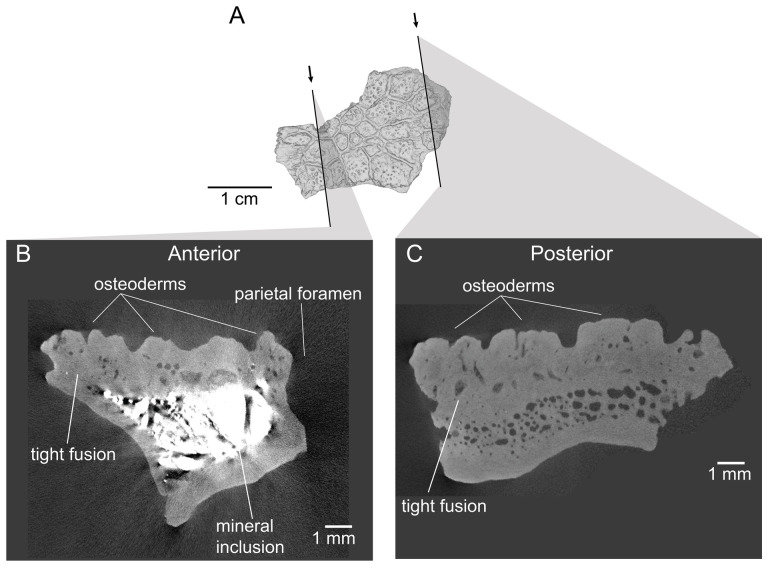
Comparisons of cranial dermal sculpturing on the parietal table of DMNH EPV.132910. (A) Rendered CT scan in dorsal view. Black lines and arrows indicate the positions and angles at which CT slices in (B) and (C) were taken. (B) Anterior slice of CT data. (C) Posterior slice of CT data.
